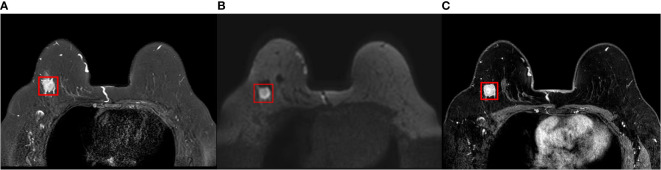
Cropping of ROI in raw MRI images of invasive breast cancer. (A) T2WI of the breast; (B) DWI of the breast; (C) Period with the most significant enhancement in DCE-MRI of the breast. ROI, region of interest; T2WI, T2-weighted imaging; DWI, diffusion weighting imaging; DCE-MRI, dynamic contrast-enhanced magnetic resonance imaging.
